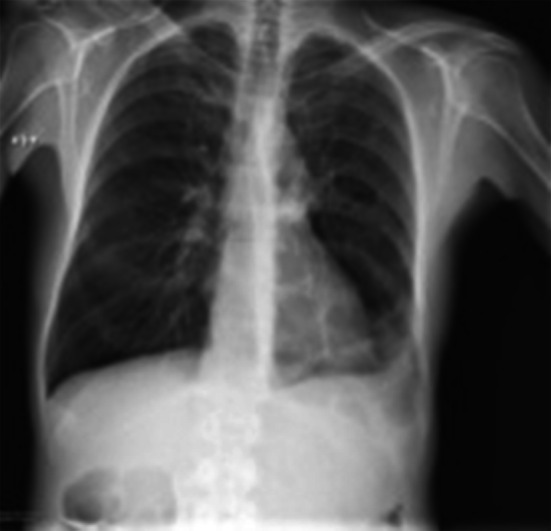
Chest radiograph of a febrile HIV-infected male (CD4 count 173 cells/μl) suggesting a *left* sided basal pneumonia
